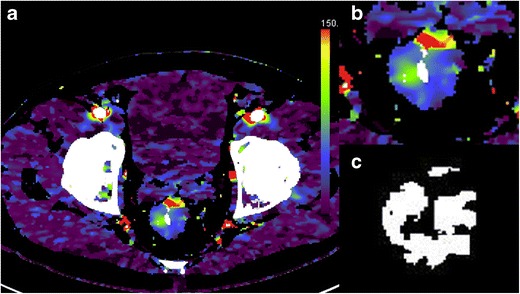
Dynamic contrast-enhanced CT (perfusion CT) blood flow parametric map (a); 2D image (b); segmented and thresholded image (c) for fractal analysis
